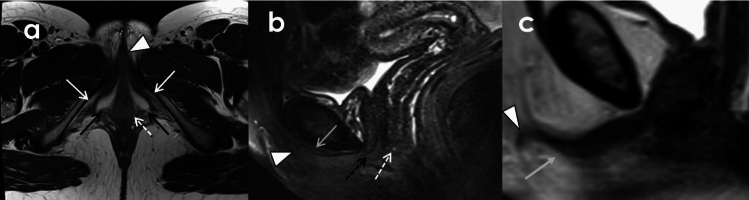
Axial T2WI (Left) and Sagittal T2WI with fat saturation (Right) demonstrating normal anatomy of the clitoris and relationship with surrounding structures in a 67-year-old female undergoing MRI for follow-up of repaired urethral diverticulum. The T2 hyperintense or intermediate vestibular bulbs of the clitoris (black arrows) flank the vaginal introitus (dashed white arrows). The T2 hyperintense crura of the clitoris run inferior to the inferior pubic rami (white arrows) and join anteriorly to form the body of the clitoris (grey arrow). The glans is ovoid in shape and intermediate in signal on T2WI (white arrow heads). The urethra (black dashed arrow) is anterior to the vaginal introitus
